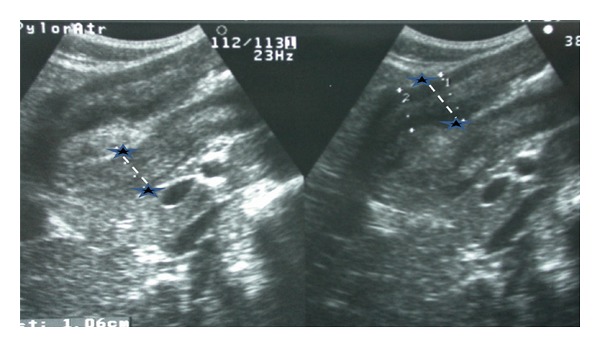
Ultrasound abdomen shows thickening of antral (asterisks) and pyloric wall.
